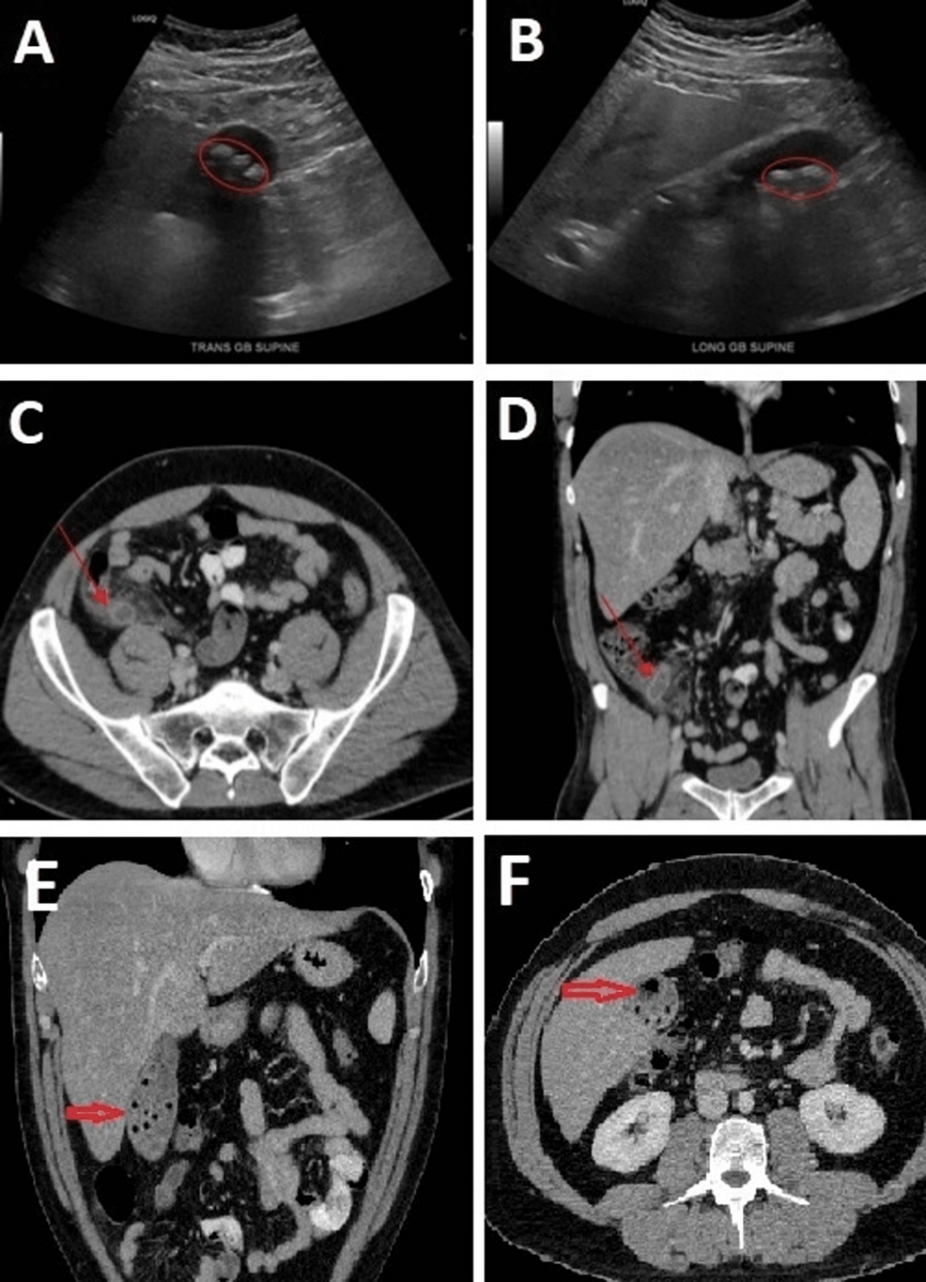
A - transverse and B - longitudinal ultrasound views of the liver and gallbladder with outlined gallbladder stones and thickened edematous wall; C – axial and D – coronal contrasted CT scan views of the abdomen and pelvis with an arrow pointing at an inflamed and distended appendix with parapedicular stranding and edema; E – coronal and F – axial contrasted CT scan images depicting a distended gallbladder with the red arrow pointing to the hyperemic wall and multiple gallstones in panel E and with the red arrow pointing to a small pocket of air, suggesting empyema of the gallbladder in panel F.
